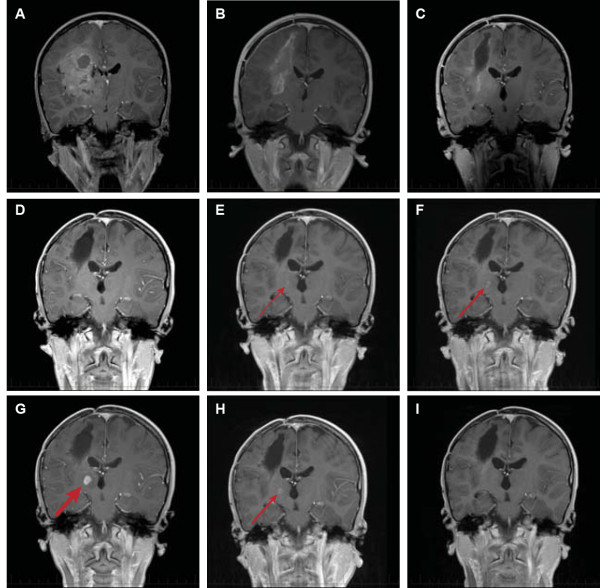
Chronological changes on magnetic resonance imaging (MRI) document the tumor recurrence and response. Coronal MRI T1-weighted images with gadolinium-based contrast were taken at the following times: (A) diagnosis, (B) post-operatively, (C) after completion of radiation therapy, (D) while receiving adjuvant chemotherapy, (E) at completion of therapy, (F) 4 months after completion of therapy, (G) upon start of vemurafenib therapy at relapse, (H) after 2 months of vemurafenib therapy, and (I) after 4 months of vemurafenib therapy.
